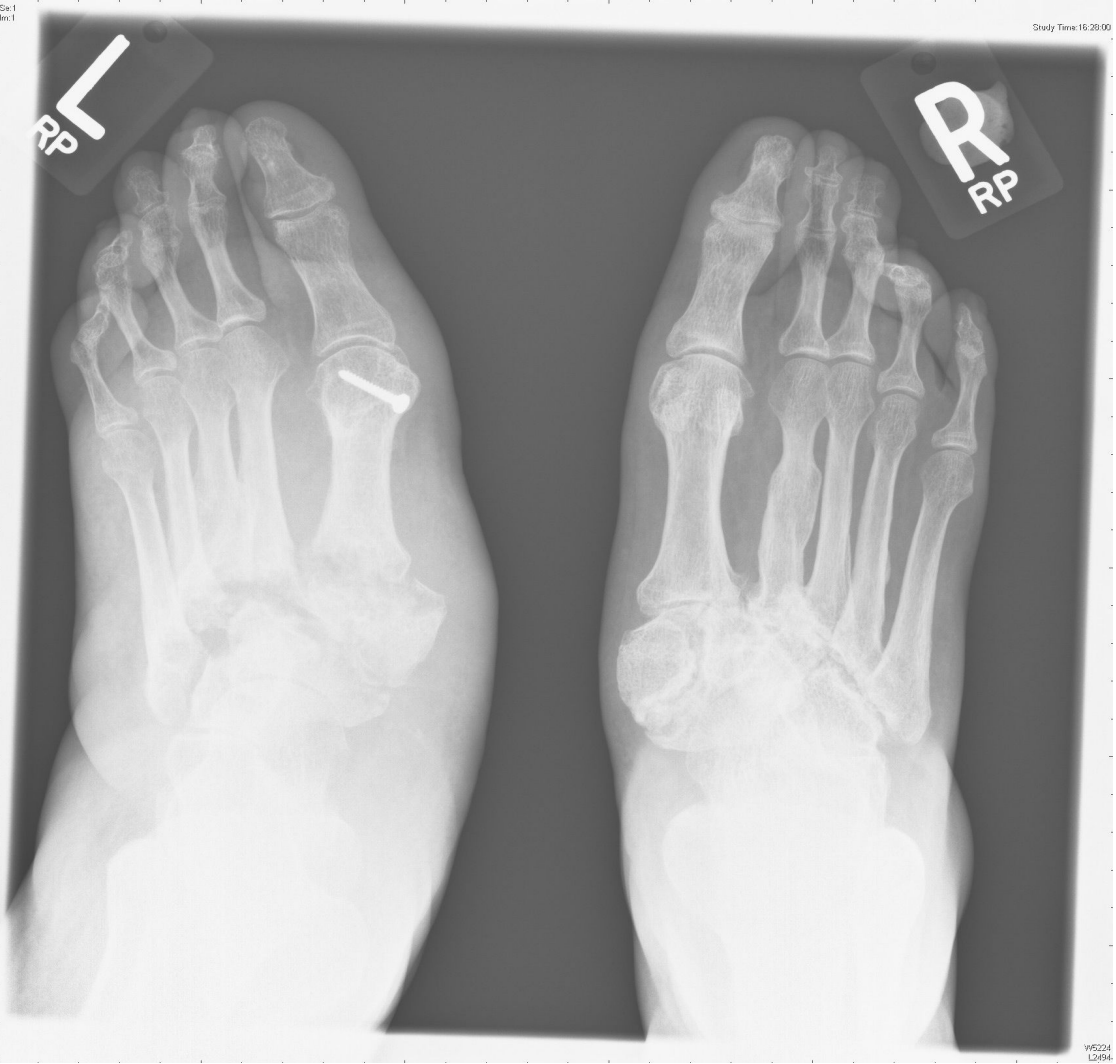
Radiograph demonstrating bilateral midfoot collapse.
